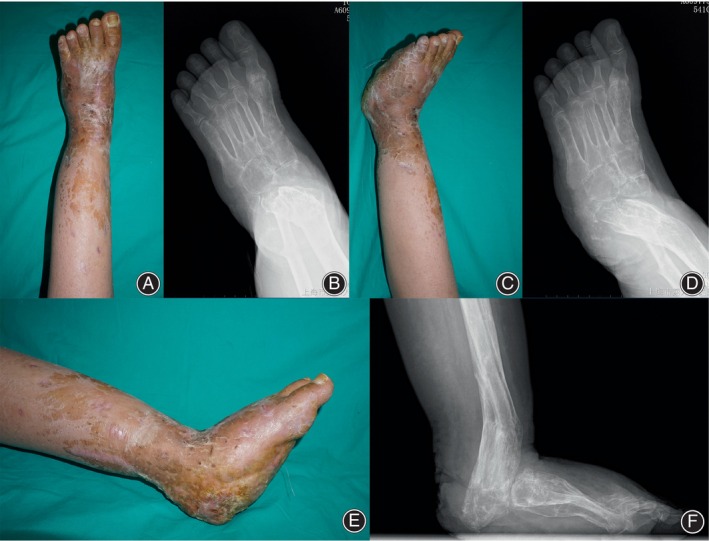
Postoperative appearance and radiography indicated satisfactory reconstruction of the defective foot and ankle. (A–D) The tibial‐calcaneal alignment was restored. (E, F) A more physiological pattern of the reproduced hindfoot bones guaranteed better weight‐bearing and ambulation.
